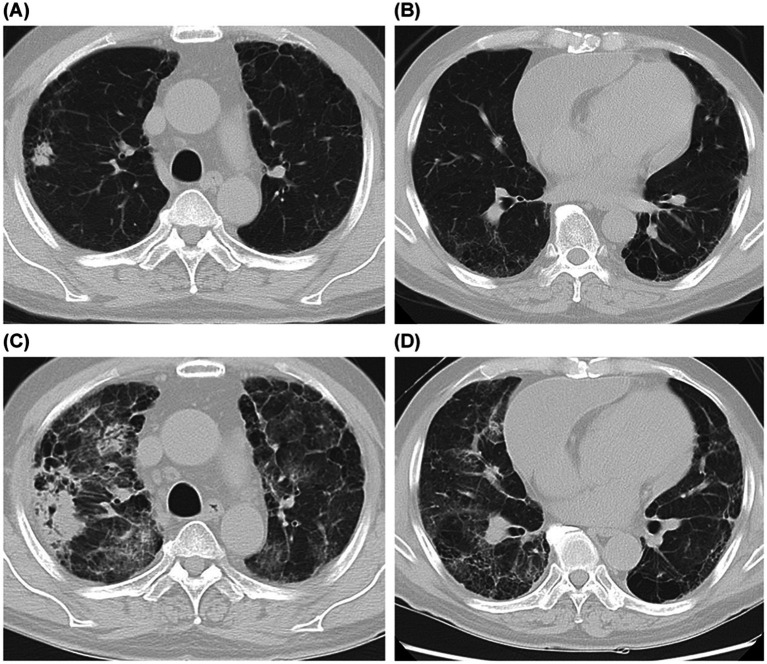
Association between pre-existing interstitial lung disease and fatal RP. (A,B) Pretreatment chest CT images showing diffuse interstitial changes in the upper and lower pulmonary fields. (C,D) CT images 14 months after SBRT indicating a severe worsening and progression of the interstitial abnormalities, diagnosed clinically as Grade 5 RP.
